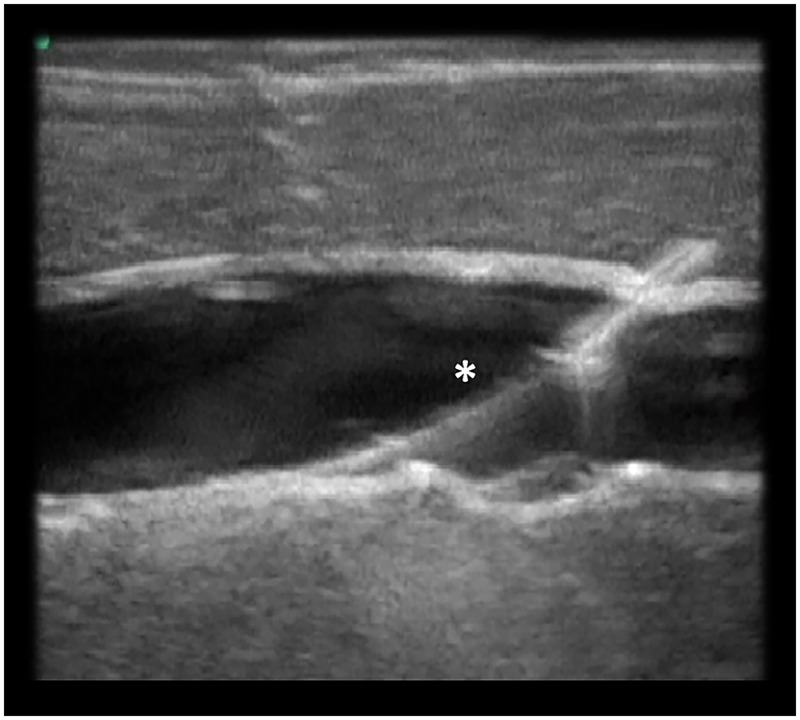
The guide-wire as visualized on ultrasonography. The guide-wire (*) is observed in the long-axis view.
